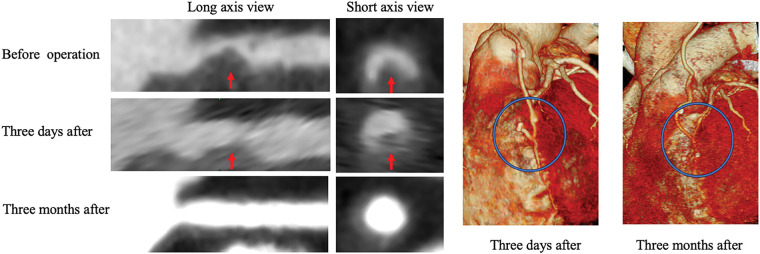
CCTA before and after the operation. Oppression of the true lumen (red arrows) gradually improved from 75% to approximately 50% at 3 days after bypass and completely disappeared at 3 months after surgery. Blue circles show LITA-LAD anastomosis site. CCTA, coronary CTA; LAD, left anterior descending artery; LITA, left internal thoracic artery
